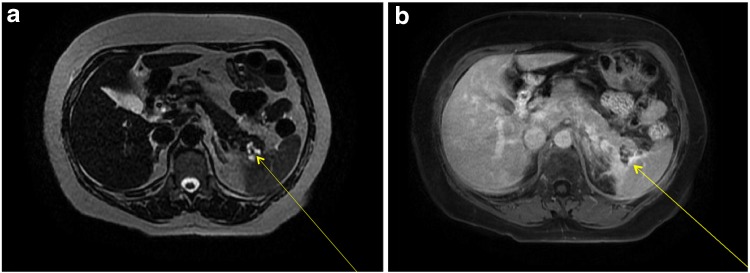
(a) T2 weighted single shot fast spin echo axial MRI displaying a mass in the pancreatic tail (yellow arrow) (b) Axial LAVA 5 min delay MRI displaying presence of a cystic mass in the pancreatic tail and peripheral postenhancement (yellow arrow). MRI, magnetic resonance imaging.
